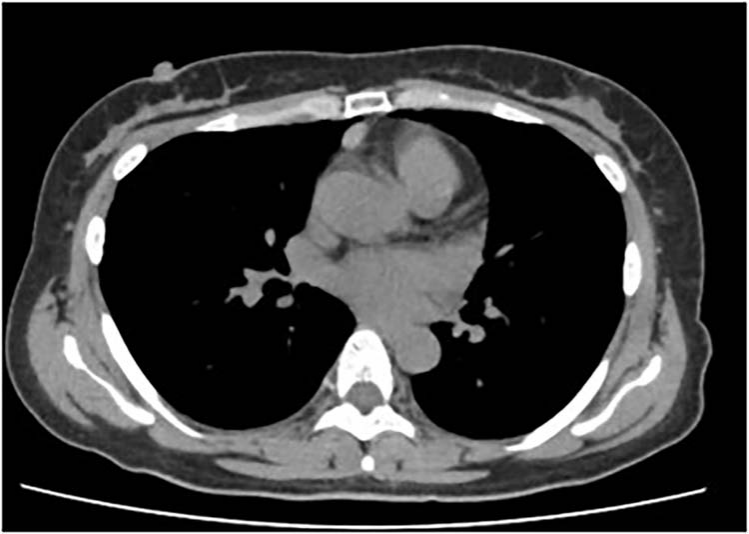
Chest CT showing a well-circumscribed tumor shadow of 15 mm in the anterior mediastinum.
